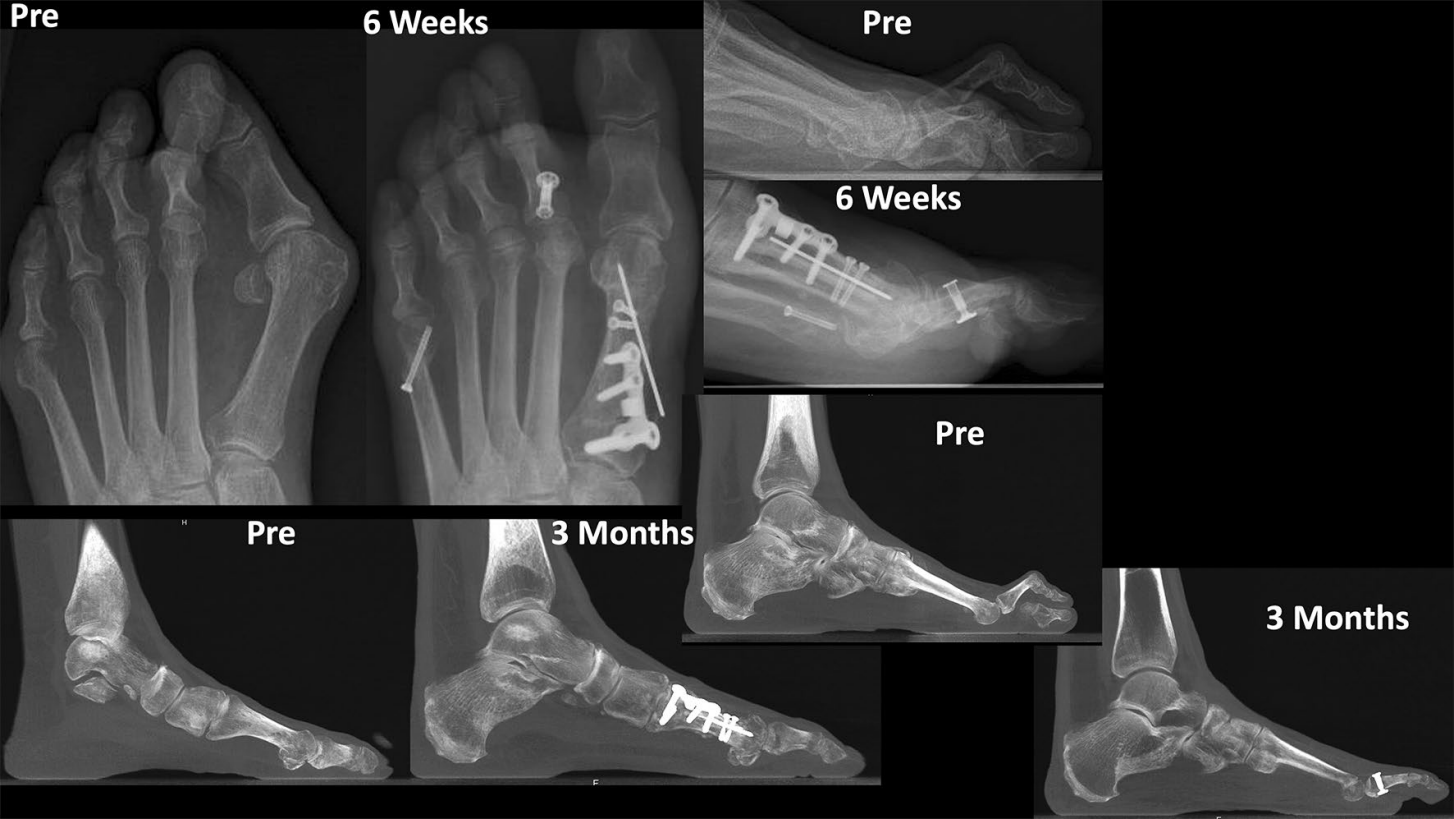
Conventional preoperative and 6-weeks postoperative weight bearing radiographic (anteroposterior and lateral views) of patient’s foot (top), as well as preoperative and 3-months postoperative wide sagittal weight bearing CT images of patient’s foot 1st and 2nd rays (bottom). Demonstration of considerable correction of the hallux valgus and bunionette deformity as well as 2nd toe deformity
